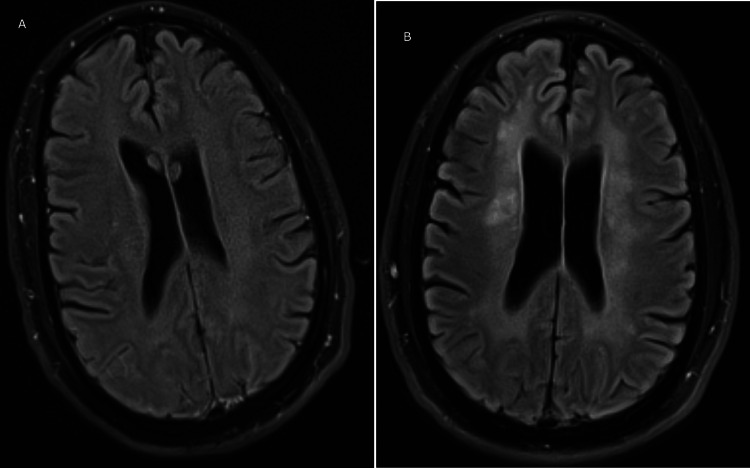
T2 FLAIR images from patient presentation and three-month follow up T2 FLAIR images of the patient at presentation and at the three-month follow-up showing evolving periventricular and deep subcortical white matter hyperintensities. A. Image at presentation; B. Image at three months.
